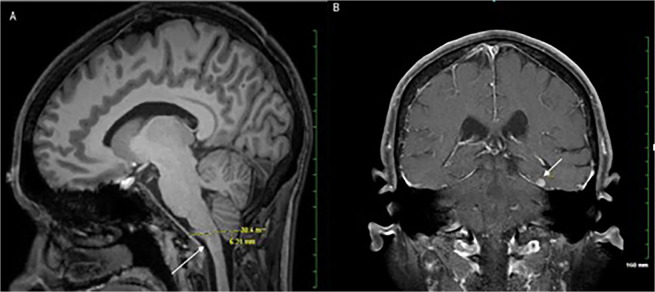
Representative Incidental Findings on Brain MRI. Arrows indicate the abnormalities in each image. Panel (A) shows low lying cerebellar tonsils (type I Chiari malformation) more than 5 mm below the level of the foramen magnum on a T1-weighted sagittal image obtained for diagnostic purposes after the original research image. A meningioma is shown on the T1-weighted coronal image in Panel (B).
